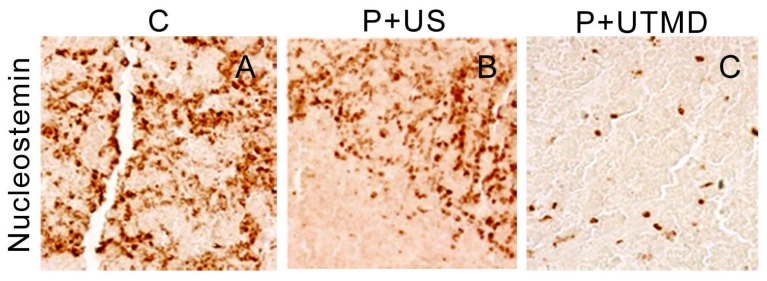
Comparison of NS gene expressions in different groups (×200). C: control group; P + US: plasmid + ultrasound irradiation group; P + UTMD: plasmid + microbubble + ultrasound irradiation group.
